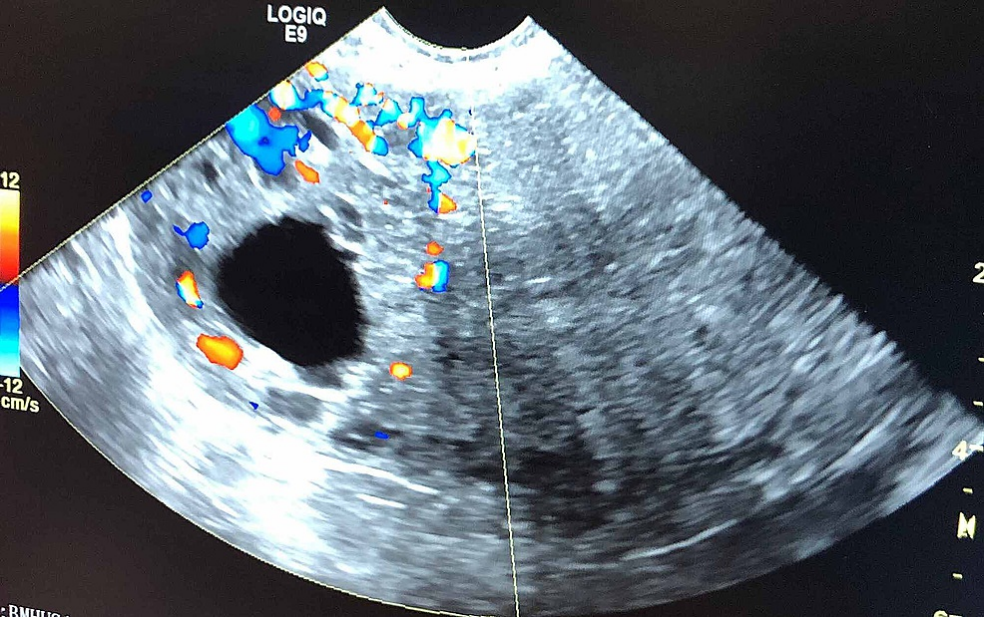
An ultrasound image of the cornual pregnancy
